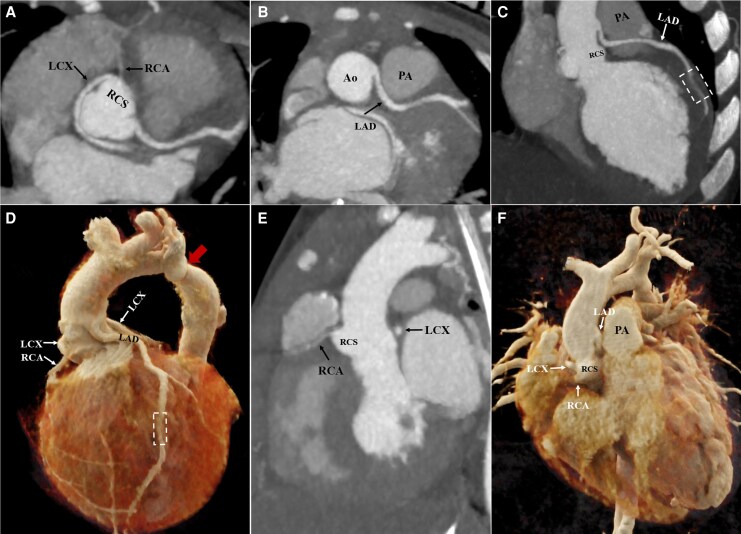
Computed tomography angiography images from a 5-month-old male infant with aortic coarctation and coronary artery anomalies. (*A–C* and *E*) Maximum intensity projection images. (*A*) Both the left circumflex artery and right coronary artery originated from the right coronary sinus. (*B* and *C*) The left anterior descending artery originated 4 mm above sinotubular junction of the right coronary sinus with an interarterial course and demonstrated a myocardial bridge in its mid-segment (dashed box). (*D* and *F*) Cinematic rendering showed coarctation of descending aorta (red arrow) along with the anomalous origin and course of coronary arteries. Ao, aorta; PA, pulmonary artery.
